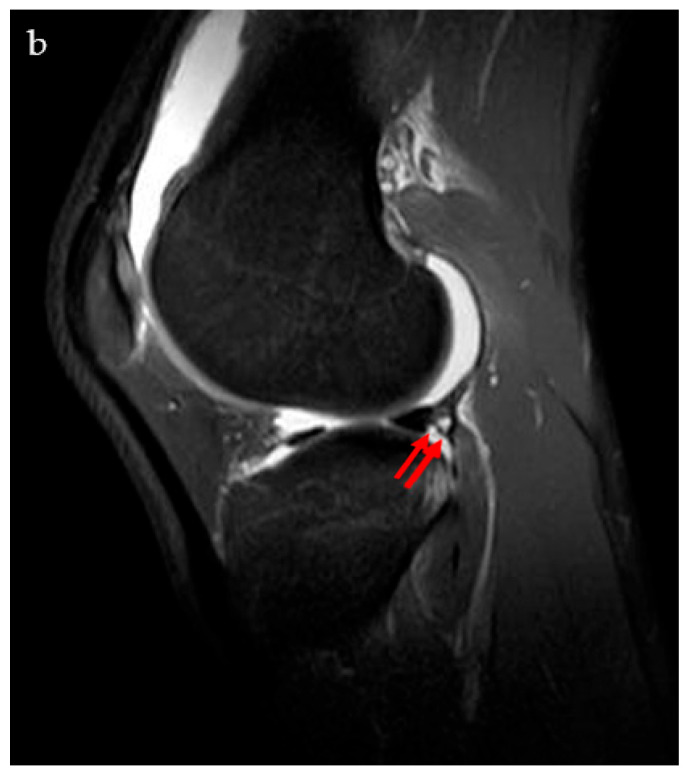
(a) Sagittal proton density-weighted (PDw) magnetic resonance (1.5 T) demonstrating the posterosuperior meniscopopliteal fascicle (psPMF) (white arrow), seen as a thin low-signal-intensity band extending from the posterior horn of the lateral meniscus to the popliteus tendon within the popliteomeniscal fascicular complex. (b) Sagittal proton density-weighted (PDw) magnetic resonance image acquired at 1.5 T showing the posteroinferior meniscopopliteal fascicle (piPMF) (red arrow), appearing as a fine hypointense band arising from the inferior edge of the posterior horn of the lateral meniscus and running obliquely toward the popliteus musculotendinous structures at the lower margin of the popliteal hiatus.
